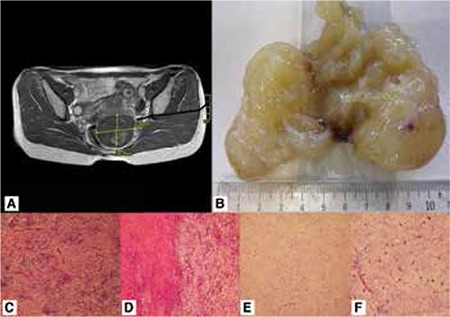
Figure 1A. MRI of the mass filling Douglas space and protruding into posterior vaginal wall
